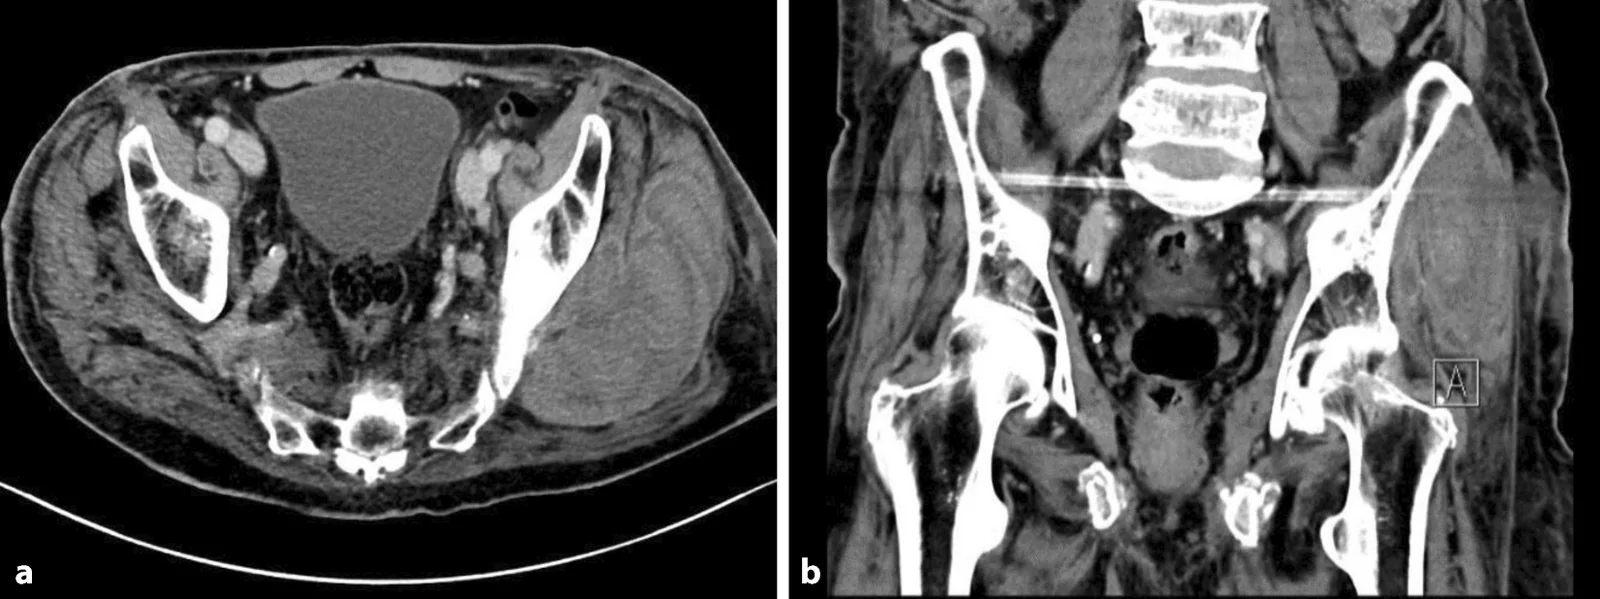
Kontrastmittelgestützte Becken-Bein-CT-Angiographie mit Darstellung eines großen glutealen Hämatoms und umschriebener Kontrastmittelaussparung sowie Nachweis eines Pseudoaneurysmas der A. glutea superior (a axial, b koronar)
